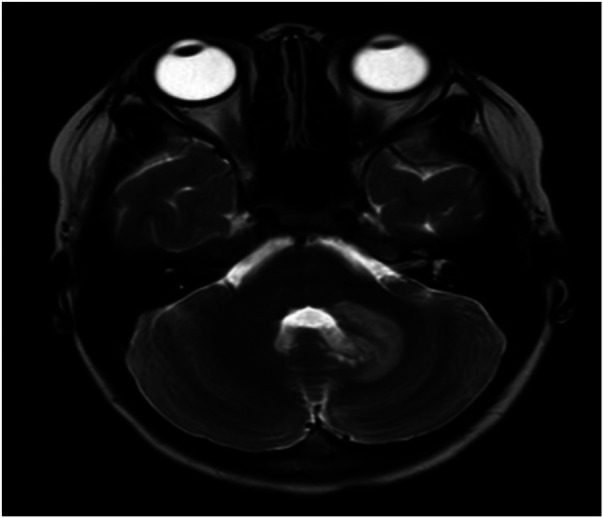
MRI T2-weighted imaging revealed an area of hyperintensity in the left cerebellar hemisphere.
